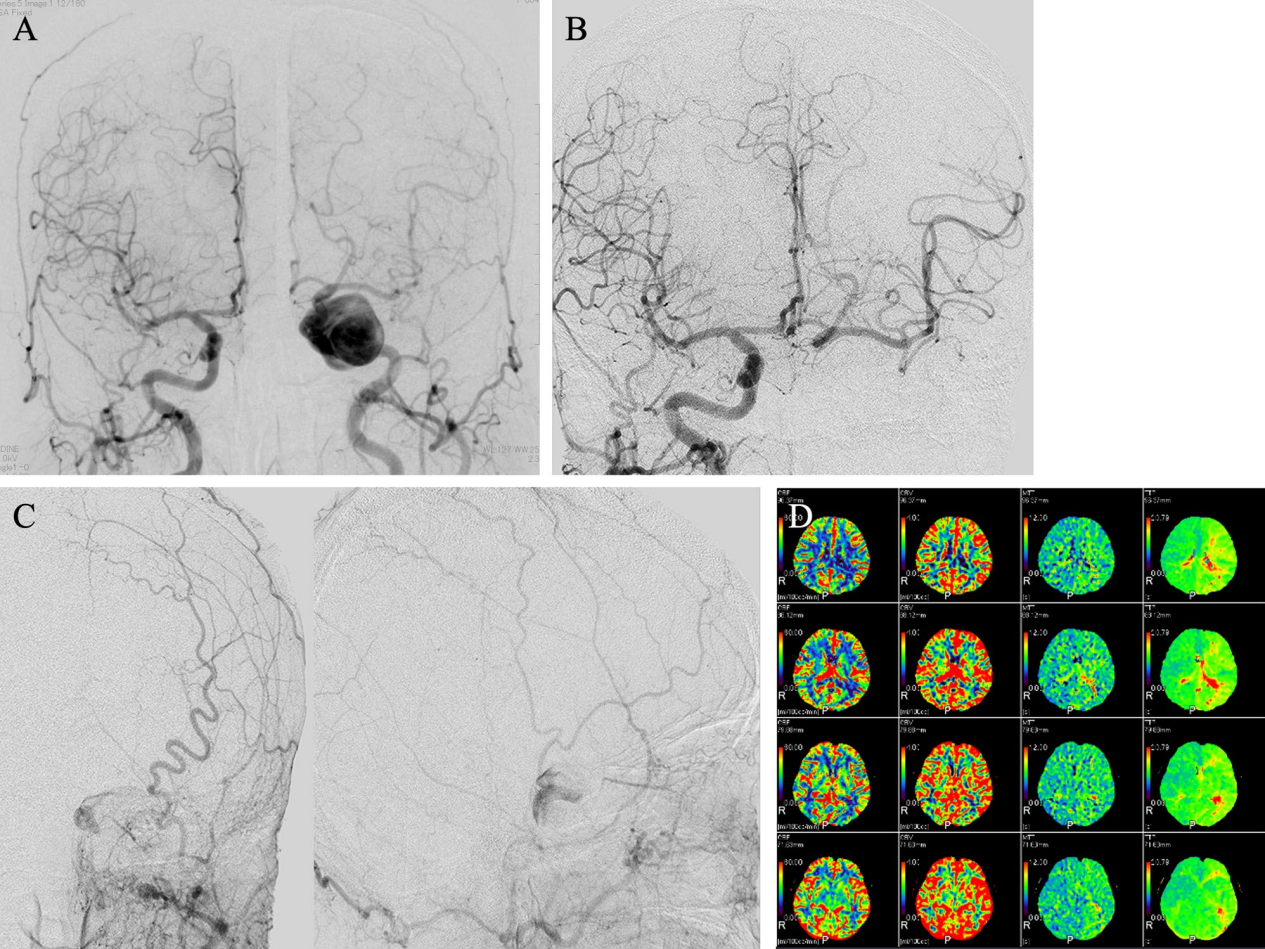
Digital subtraction angiography (DSA) and balloon test occlusion test of the left internal carotid artery (ICA). (a) Anterior–posterior (AP) view of the left and right common carotid artery angiograms. (b) BTO of the left ICA demonstrating collateral flow through the anterior communicating artery. (c) AP and lateral views of concurrent injection of the left external carotid artery showing retrograde flow to the aneurysm via the ophthalmic artery. (d) Perfusion computed tomography with BTO. Cerebral blood flow, cerebral blood volume, mean transit time, and time to peak are shown from left to right.
